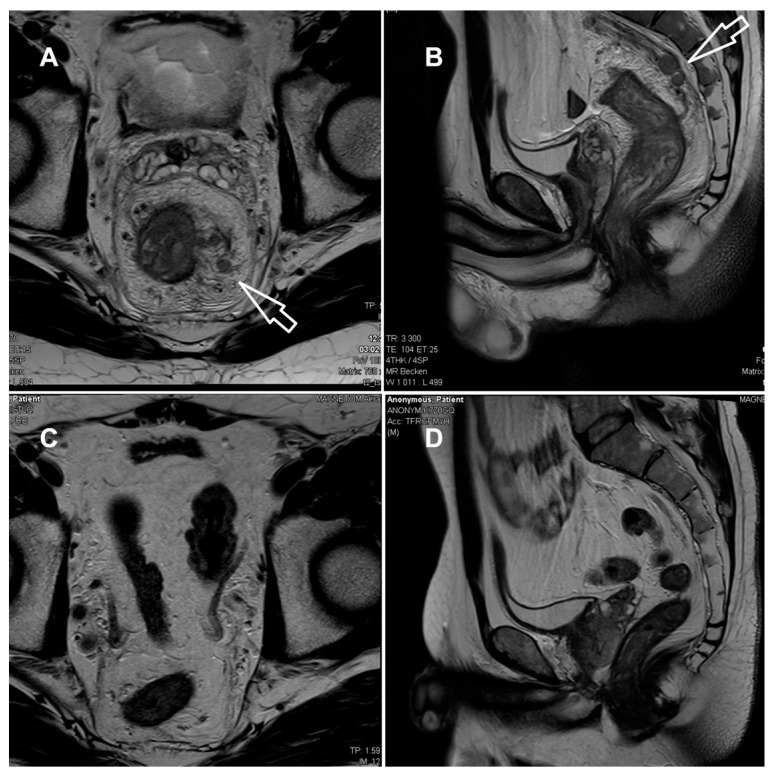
MRI of the rectum at presentation (A,B) and after 3 months (C,D). (A,B) T2-weighted axial (A) and sagittal (B) at initial presentation, showing long-segment, circumferential thickening of the rectal wall (white arrows) with marked mural irregularities, edema, perineural and perivascular infiltration; multiple lymph nodes with irregular shape within and beyond the mesorectal fascia, extending to the external iliac region. Infiltration of the mesorectal fascia and peritoneum with mucosal diffusion restriction. Mild pelvic ascites. Suspected colorectal carcinoma staged as T4a N2b M1 MRF+ EMVI+. (C,D) After antibiotic treatment, complete regression of the rectal wall and mesorectal fascia thickening; regression of edema and no lymphadenopathy. (MRF = mesorectal fascia, EMVI = extramural vascular invasion).
